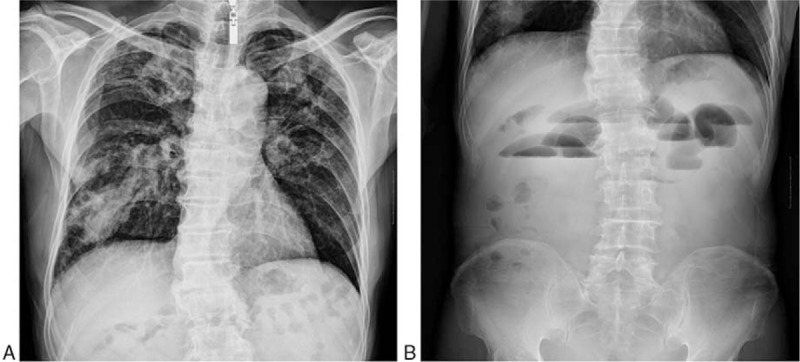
Plain x-rays of chest and abdomen on hospital admission. (A) Chest x-ray showing bilaterally patchy infiltrates, increased bronchovascular markings, and mass in the right lower lung fields. (B) Abdomen x-ray showing showed the gaseous distention of the bowel with air-fluid levels.
